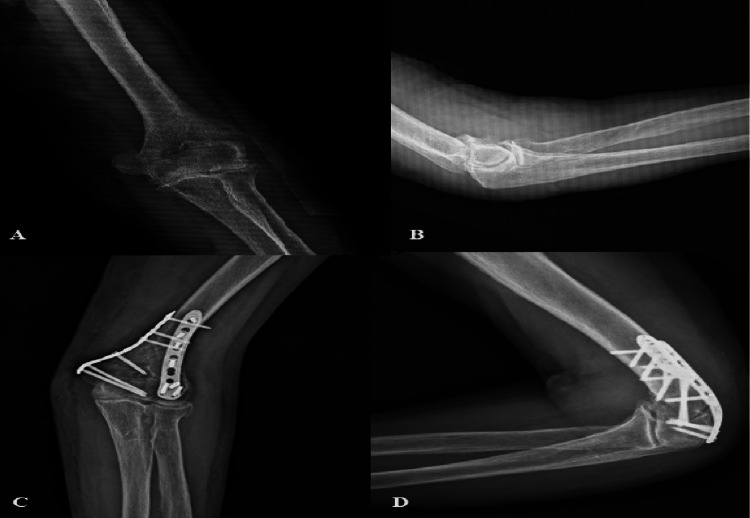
78-year-old male with a displaced low transcondylar fracture (AO/OTA 13A2-3) (A) Preoperative anterioposterior and (B) lateral radiographs of a 78-year-old male patient with a displaced low transcondylar fracture. (C, D) Postoperative anteroposterior and lateral radiographs showed a stable fixation with an orthogonal pattern before the completion of follow-up
